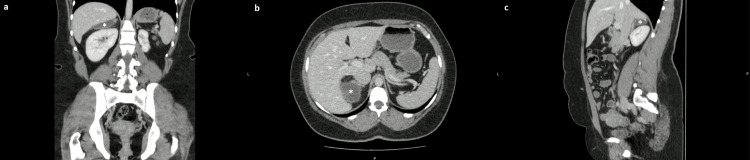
Abdominal CT performed after the percutaneous drainage and sclerosis of the cystic lesion: cystic lesion (*) with somewhat lobulated contours, without solid components adjacent to the upper pole of the right kidney, the right adrenal gland, and the lower surface of the right hepatic lobe, measuring 58 × 34 × 14 mm. (a) Coronal section. (b) Axial section. (c) Right parasagittal section. CT: computed tomography
